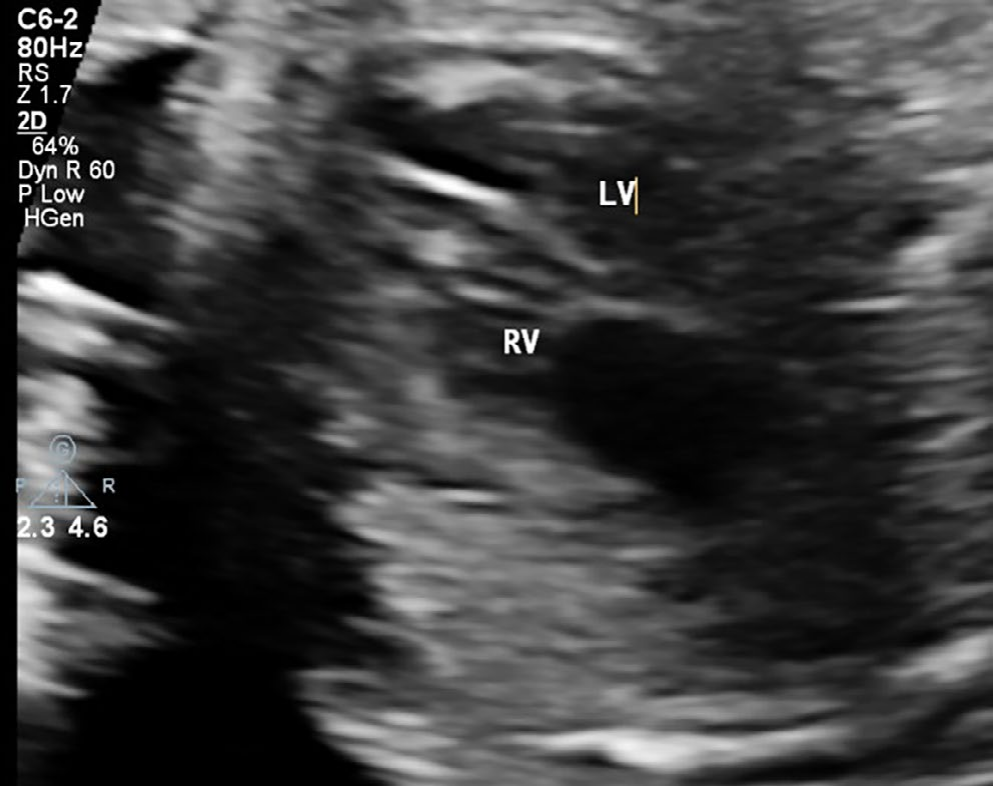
Axial fetal ultrasound demonstrates normalization of right ventricular size and resolution of the previously noted size discrepancy between the right ventricle (RV) and left ventricle (LV), with reversal of the disproportion.
